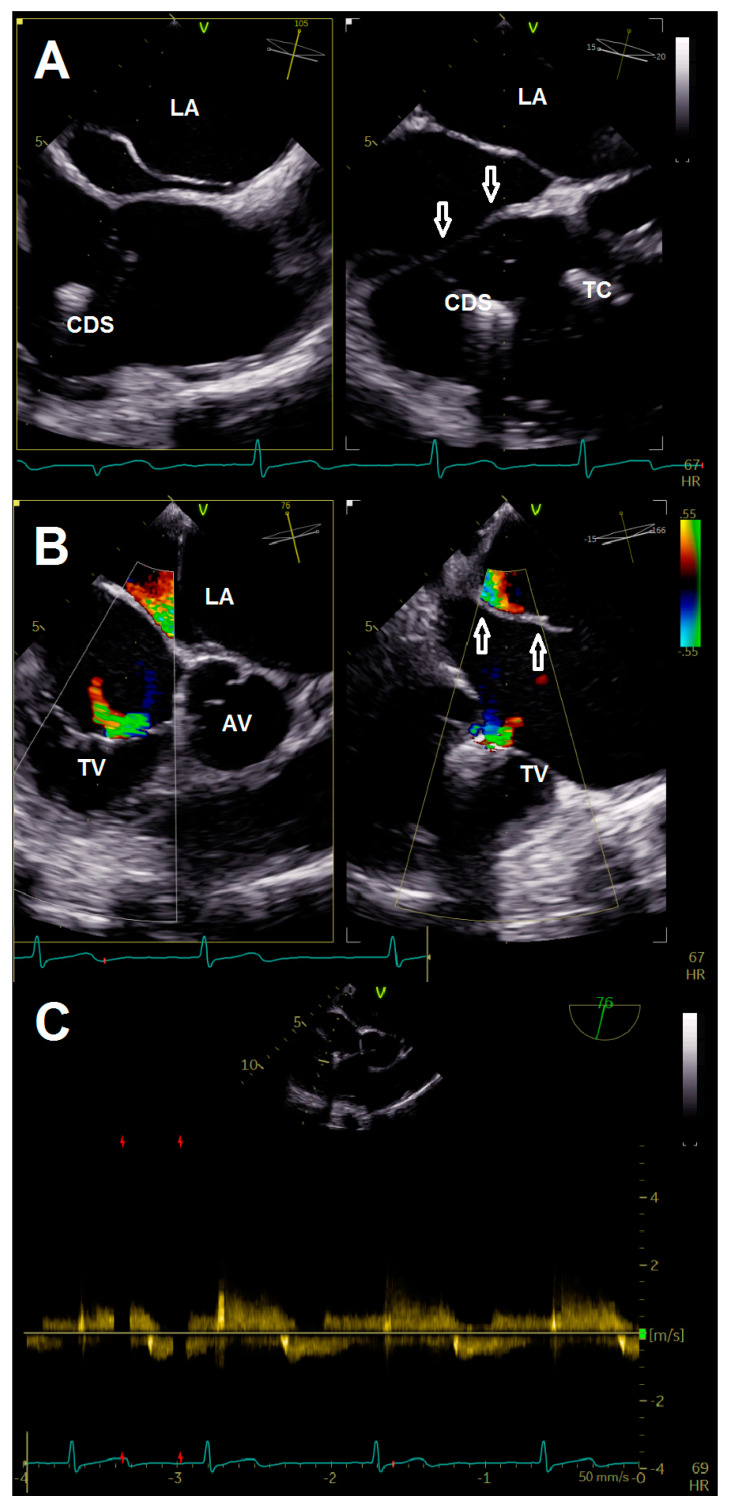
(A) Crossing of membrane (arrows) during introduction of the clip delivery system. (B) Multiplane color doppler evaluation of tricuspid regurgitation after implantation of three clips. (C) Continuous wave doppler after clip implantation showing no relevant tricuspid stenosis. Abbreviations: AV, aortic valve; CDS, clip delivery system; LA, left atrium; TC, TriClip^®^; TV, tricuspid valve.
